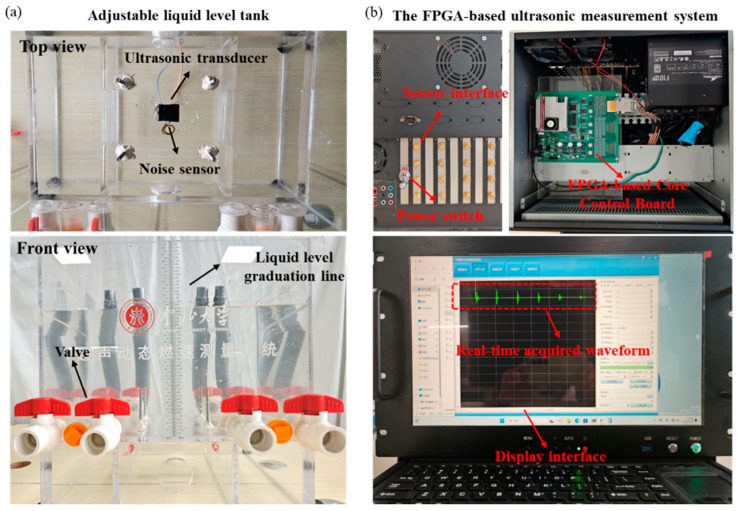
Experimental testing system. (a) Adjustable liquid level tank. (b) The FPGA-based ultrasonic measurement system.
